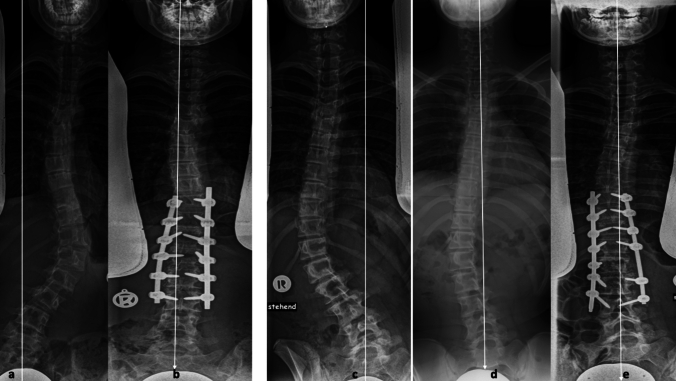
Two examples of posterior correction of thoracolumbar curves. In both cases, the EV was chosen as LIV although it was neither a stable vertebra nor LSTV (a, c). In the first case, L3 is the lower EV while L4 is the LSTV; T10 is the upper EV (a). Fusion was done form T10 (upper EV) to L3 (lower EV—in this case LSTV-1) (b). In the second case, L3 is the lower EV while L4 is the LSTV; T8 is the upper EV (c). The traction radiograph (d) demonstrates that the EV can move in the stable zone; L3 has now become the LSTV (instead of the L4 on the normal AP film). Fusion was done from T9 (EV -1) to L3 (lower EV—in this case LSTV-1 on the normal AP film, but LSTV on the Traction film) (e)
